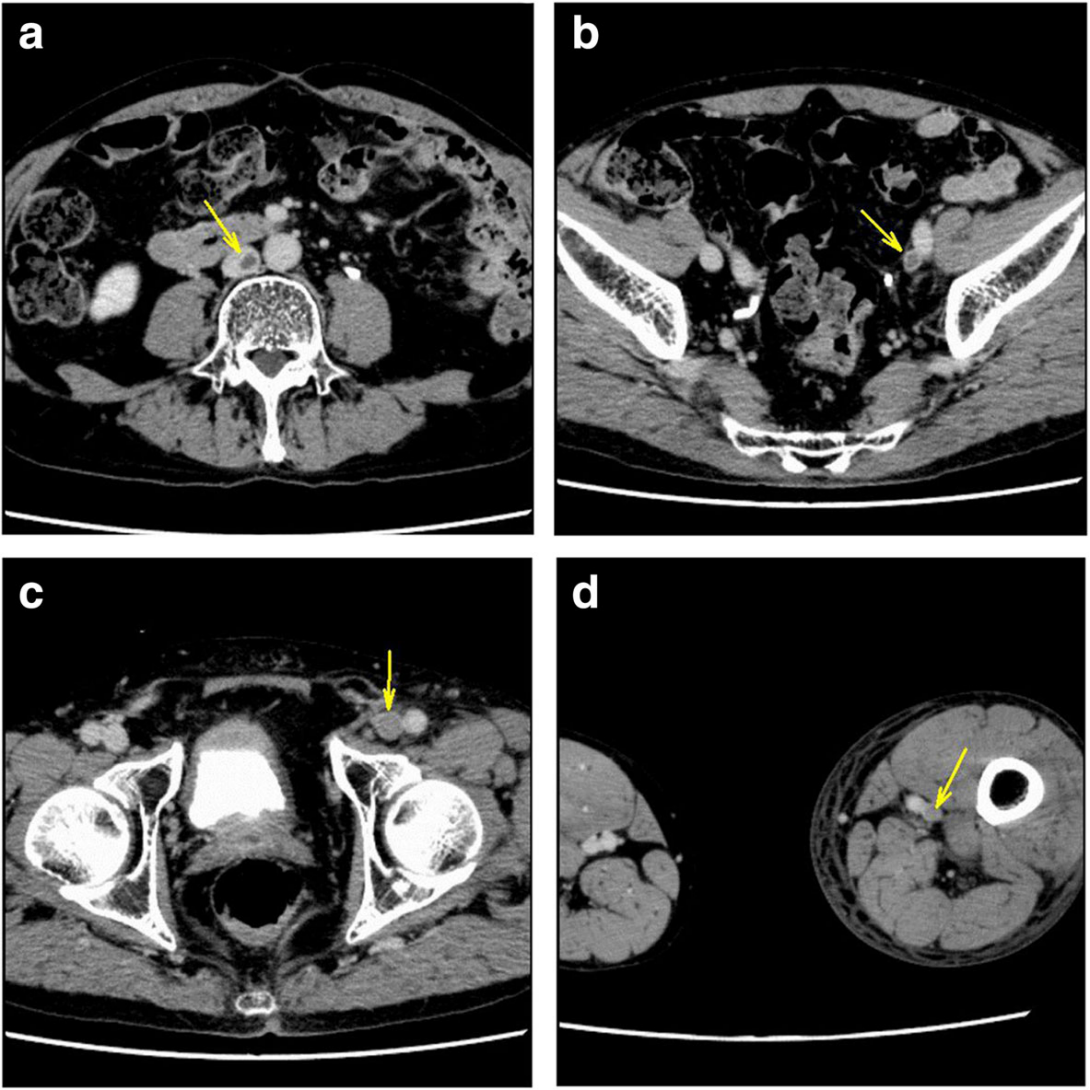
Contrast-enhanced computed tomography on the first visit. A contrast deficit in (a) the inferior vena cava at the level of kidney lower lobe (the yellow arrow), (b) the left external iliac vein (the yellow arrow), (c) the left superficial femoral vein (the yellow arrow), (d) the left popliteal vein (the yellow arrow)
